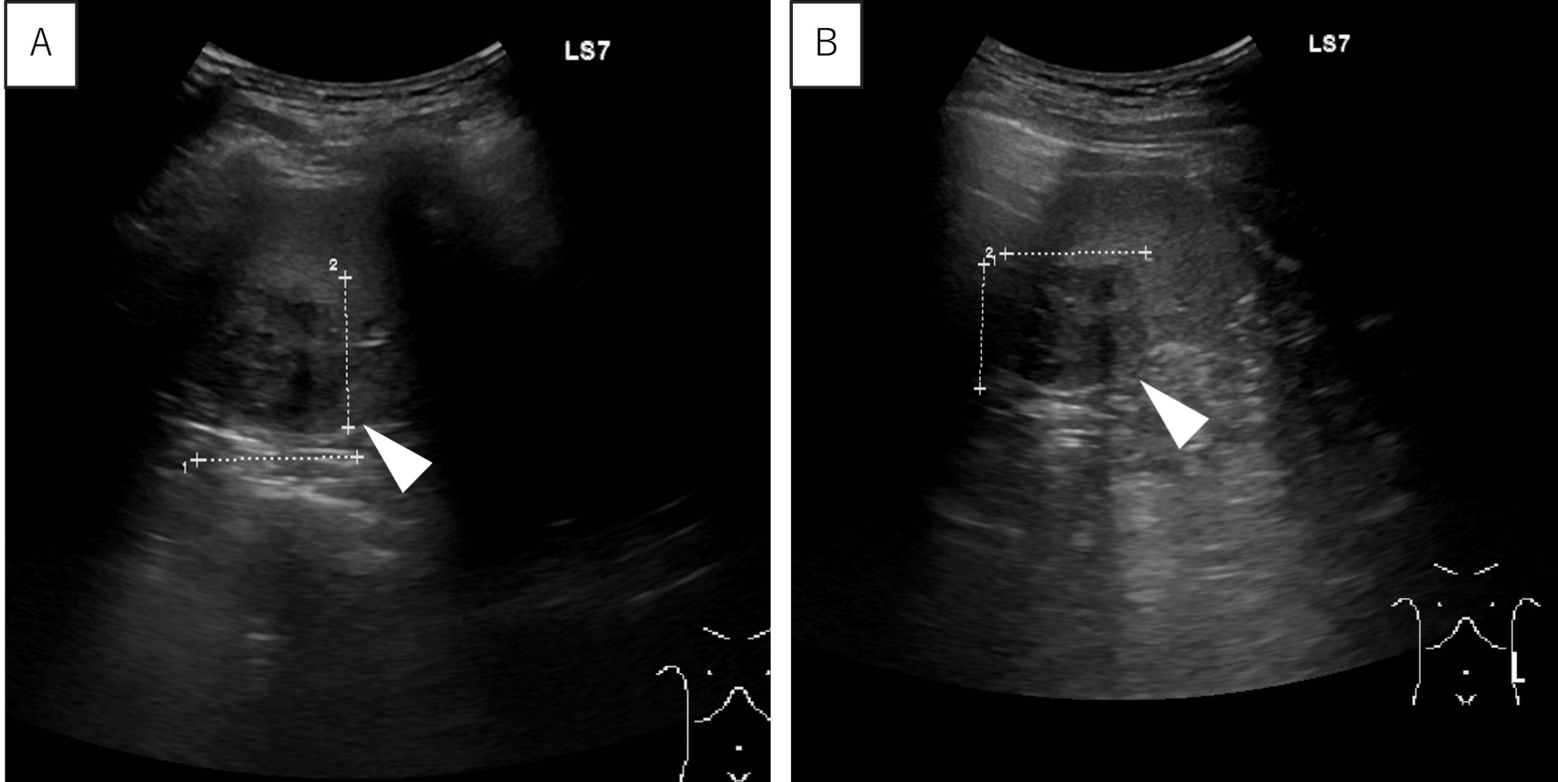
Abdominal ultrasonography Abdominal ultrasonography showing well-circumscribed hypoechoic masses in the spleen. A: Transverse view, B: Longitudinal view
